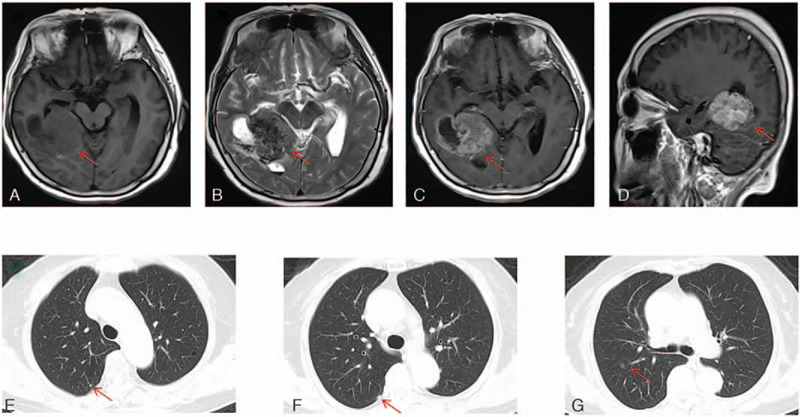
The cancerous mass in intracranial MRI. (A)T1WI, (B)T2WI, (C-D)Enhanced imaging. (E-G) The pre-operative chest images showed multiple solid and ground glass nodules in the upper right lung. MRI = magnetic resonance imaging.
